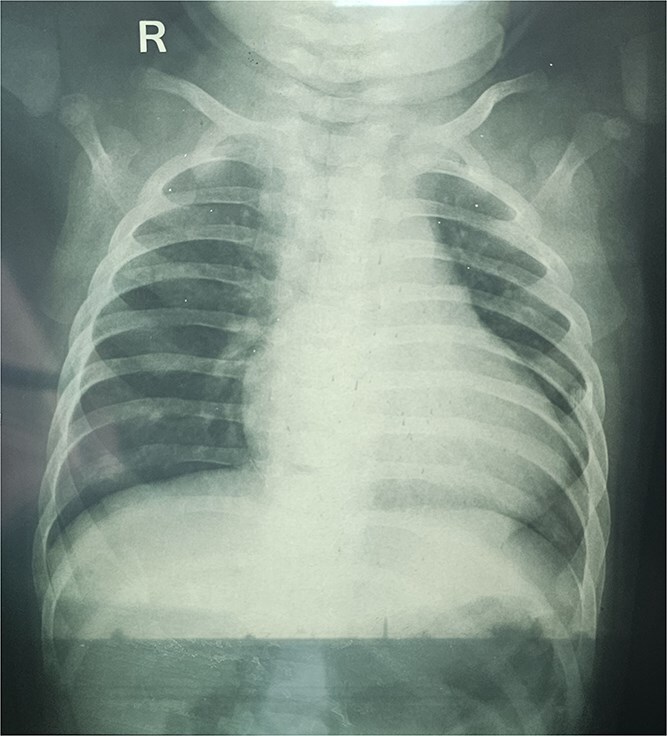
Chest radiograph shows mild cardiomegaly with decreased pulmonary vascular markings and clear lung fields.
